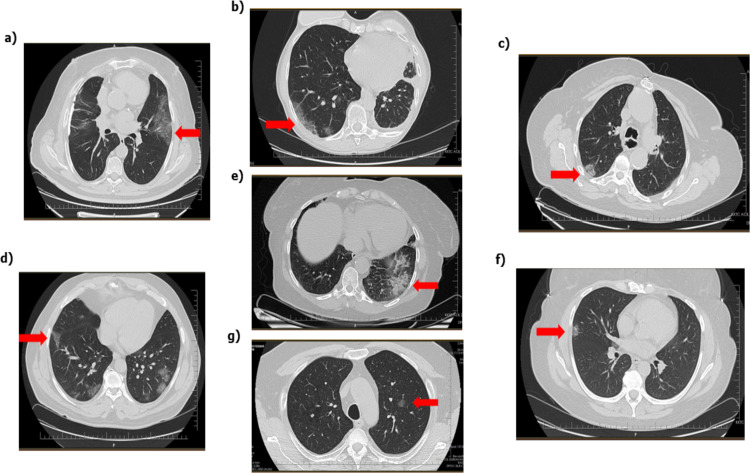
Various lesions of COVID-19 RT-PCR positive patients whose CT images were retrospectively analyzed. a) Ground-glass images of the left lung with lingula septal thickening and air bronchograms b) Ground-glass images of the right lung with the sub-pleural location at the base and involvements accompanied by septal thickenings. c) Ground-glass images of peripheral subpleural and peribronchovascular in the upper lobe of the right lung. d) Central focal ground-glass view in the apicoposterior segment of the left lung upper lobe. e) Cobblestone images in the left lung basal. f) Ground-glass densities accompanied by septal thickenings with widespread involvement in the lower lobe and right middle lobe of both lungs. g) Consolidation area with peripheral involvement in the right lung middle lateral segment
